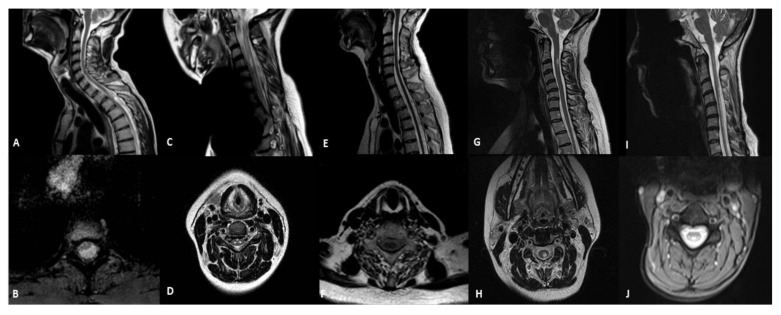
Sagittal and axial T2-weithing images of MRI in patients with mitochondrial diseases and spinal cord involvement. (A,B) MIDD with m.3243A > G mutation (patient 1): selective thinning of the spinal cord with a cystic-like lesion located in the white matter of dorsal and lateral columns; (C,D) Leigh syndrome with m.13513G > A (patient 2): hyperintensity spinal grey matter in the cervical and the upper-thoracic spinal cord segments and medullary atrophy; (E,F) ANS with *POLG* mutation (patient 3): medullary atrophy without signal alterations; (G,H) LHON with m.11778G > A (patient 4): focal hyperintensity in the cervical posterior columns; (I,J) LBSL with *DARS2* mutation (patient 5): abnormalities of the T2 signal of the cervical and thoracic spinal cord involving dorsal columns and lateral corticospinal tracts.
